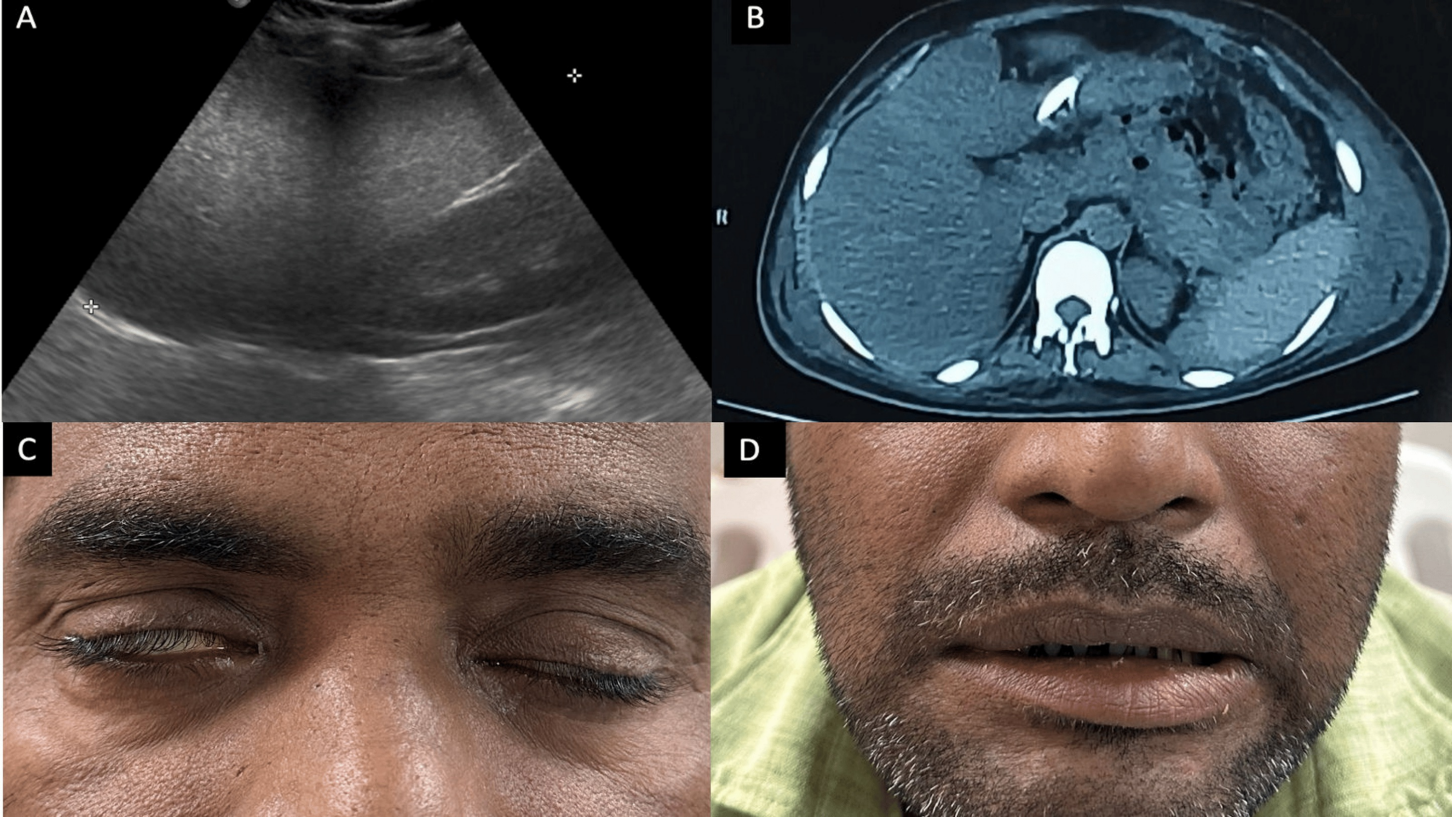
Extrahepatic manifestations of acute viral hepatitis E Figure A: Ultrasonography abdomen showing hepatomegaly. Figure B: CT of the abdomen showing bulky pancreas with peri-pancreatic fat standing suggestive of acute pancreatitis. Figure C: Figure showing Bell's phenomenon. Figure D: Facial asymmetry, depicting the features of tight-sided lower motor neuron type 7th cranial nerve palsy features CT: computed tomography
